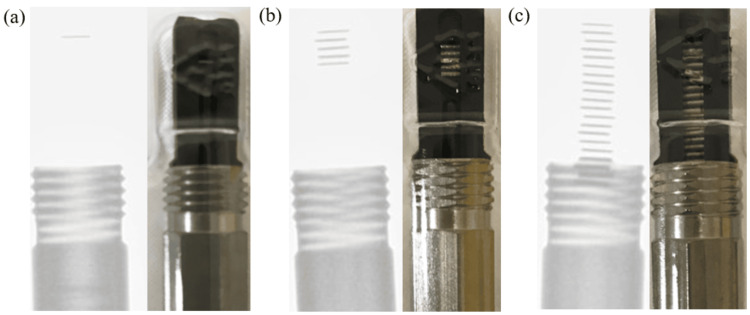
X-ray fluoroscopy image of a blister pack. Figure 4 shows X-ray images of blister packs containing (a) one unit, (b) five units, and (c) 20 units. As seen in the figure, the position of the radiation source varies depending on the number of units. In the case of five units, all sources are located within the plastic portion.
